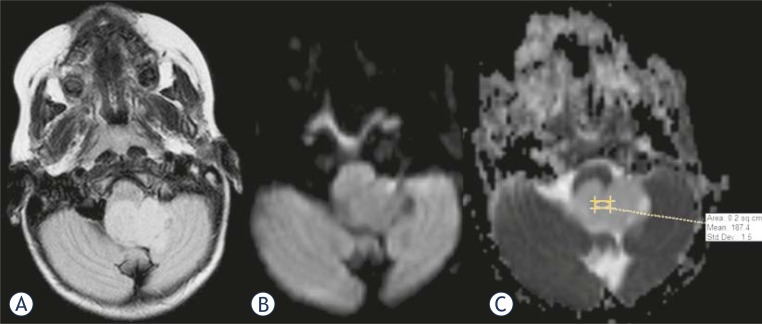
MR images in axial plane of a patient with pilocytic astrocytoma. A: Fluid-attenuated inversion recovery (FLAIR) image with a homogenous, slightly hyperintense tumour in the fourth ventricle and left foramen of Luschka. Diffusion-weighted image (B), and map of apparent diffusion coefficient (ADC) (C) without restriction of the diffusion. Intratumoural diffusion coefficient is 187.4 × 10^−5^ mm^2^/s.
